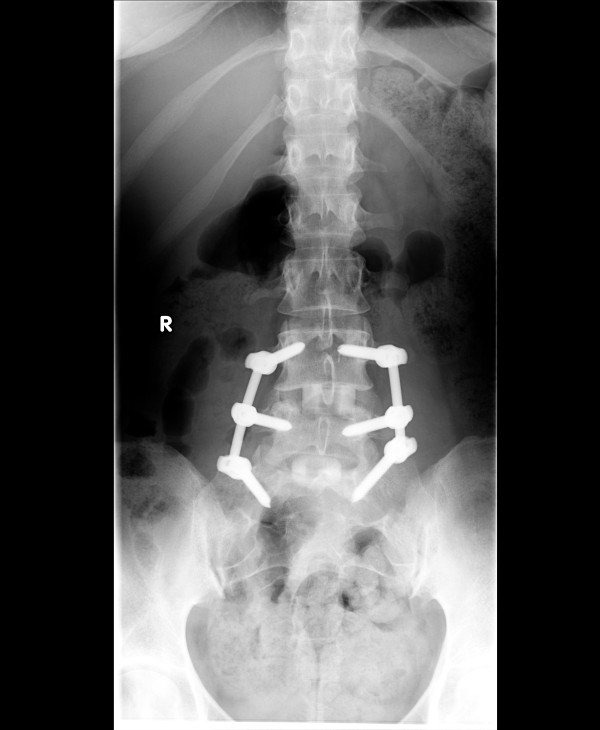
AP X-ray shows L4 to S1 instrumented fusion using minimally invasive approach. In order to perform this type of surgery, the location of skin incision must be very precise.
